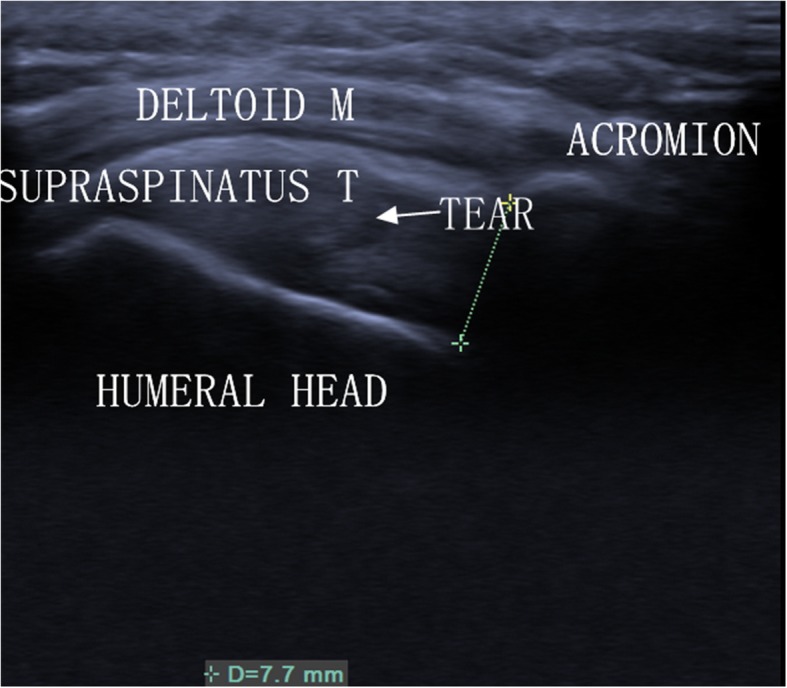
Ultrasound image of AHD in a shoulder with a partial-thickness tear of the supraspinatus tendon. T = tendon; M = muscle
